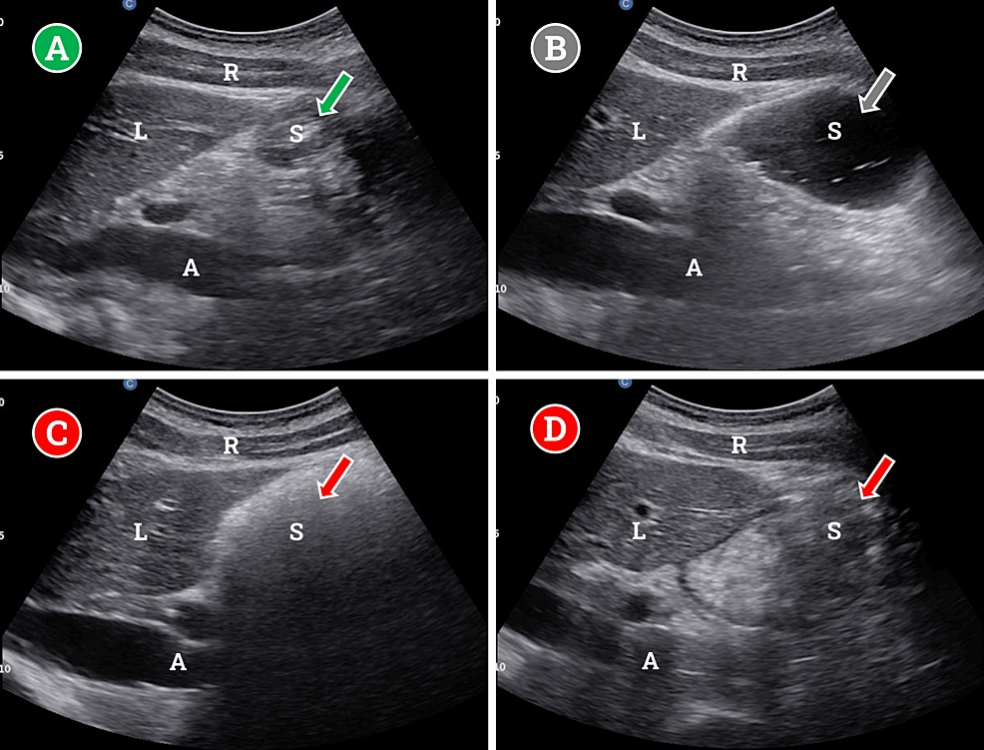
Gastric ultrasound findings based on different types of gastric contents. (A) Target-like appearance of the empty stomach (flat antrum), low risk of aspiration; (B) dilated stomach with liquid contents, volume assessment required; (C) stomach with solid contents and air creating artifact, early phase following eating, high risk of aspiration; (D) dilated stomach with hypoechoic components, later phase following solid or non-clear liquid consumption. R: rectus abdominis muscle; L: liver; S: stomach (antrum); A: aorta Images were taken and generated by the authors for the purpose of publishing in this journal only.
